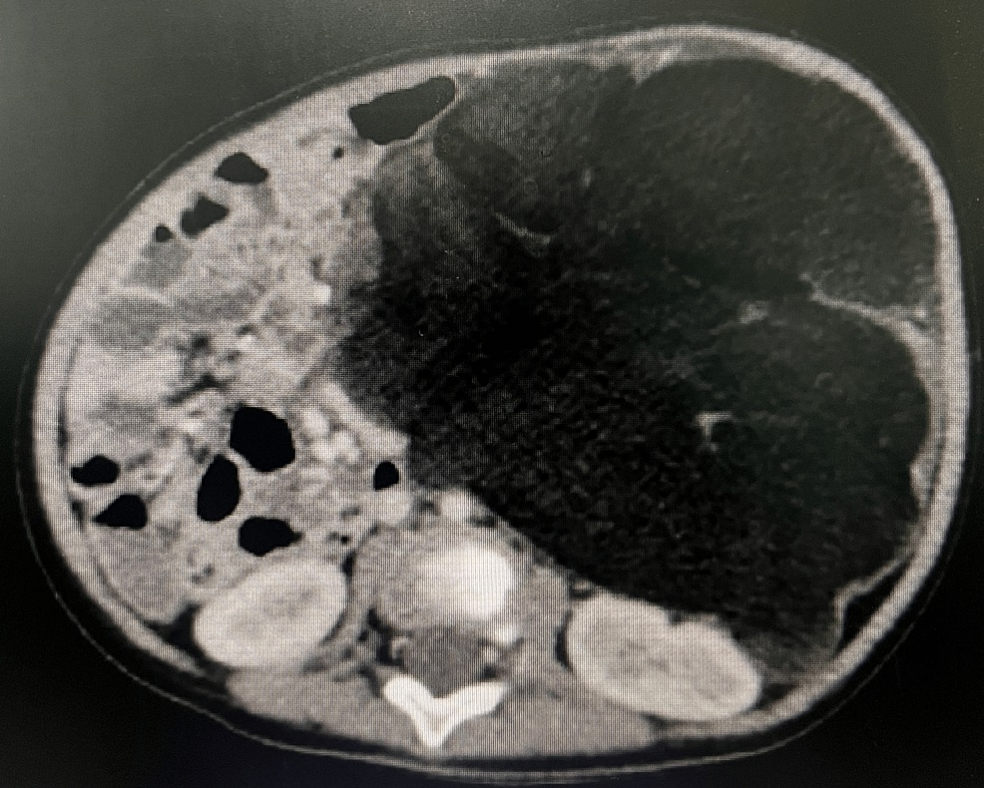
Abdominal CT scan of the patient Seen is a large intraperitoneal multilobulated mass with a fat component in the anterior of the left kidney
